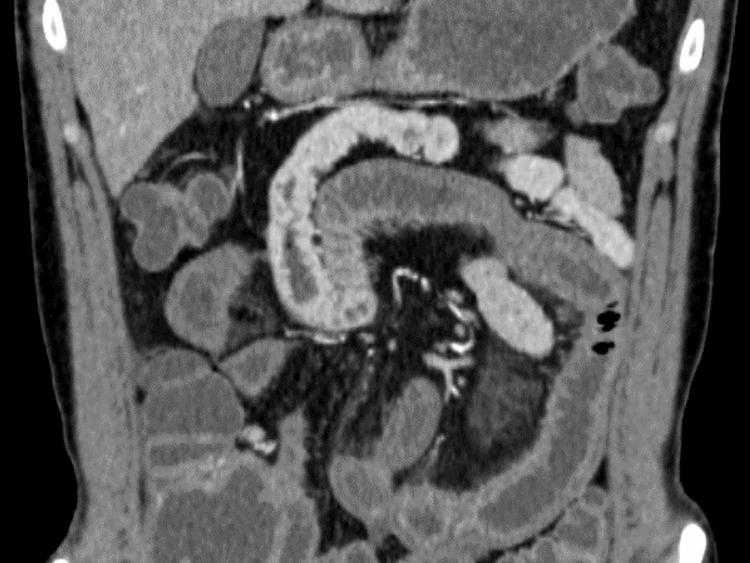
Abdominal computed tomography with contrast shows extensive edematous wall thickening of the small intestine
